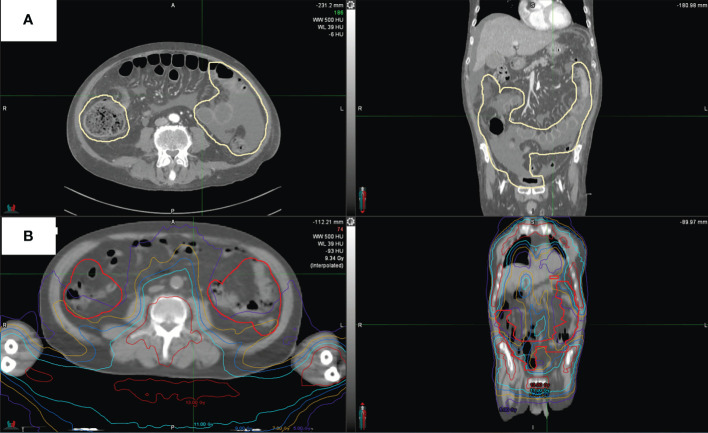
CT scan of one representative patient. (A) shows intestinal areas with aGvHD as outlined in pale yellow. (B) shows aGvHD as contoured on this patient’s original treatment plan and dose distribution.
